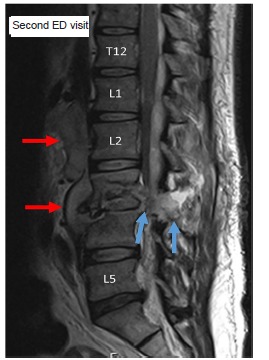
Midline sagittal magnetic resonance image taken on second visit re-demonstrates epidural abscess (red arrows) that has spread to extensively involve posterior vertebral soft tissue elements (blue arrows). Lumbar vertebrae 1 (L1), 2 (L2), and 5 (L5). Thoracic vertebrae 12 (T12). *ED*, emergency department.
